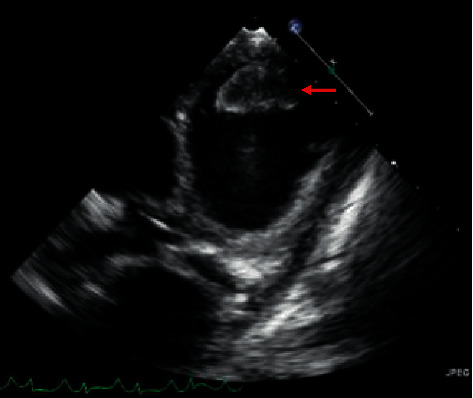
Modified parasternal short-axis view revealing a 2.7 × 1.3 cm thrombus in the left ventricle.
